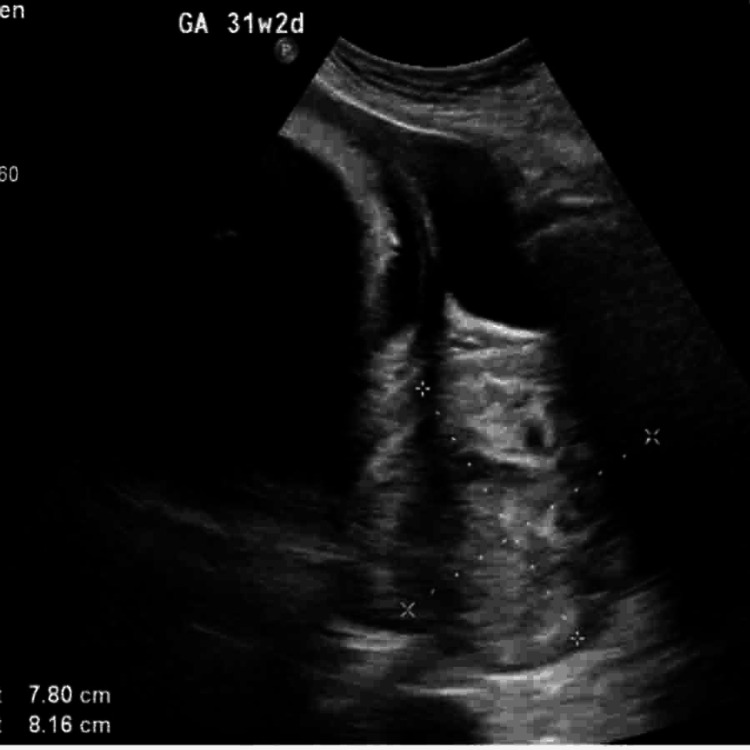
Ultrasound at 31 weeks and 2 days of gestation showing a large cervical fibroid measuring approximately 7.80×8.16 cm. The fibroid appears as a heterogeneous, hypoechoic mass arising from the lower uterine segment, potentially impacting cervical anatomy and influencing delivery planning.
